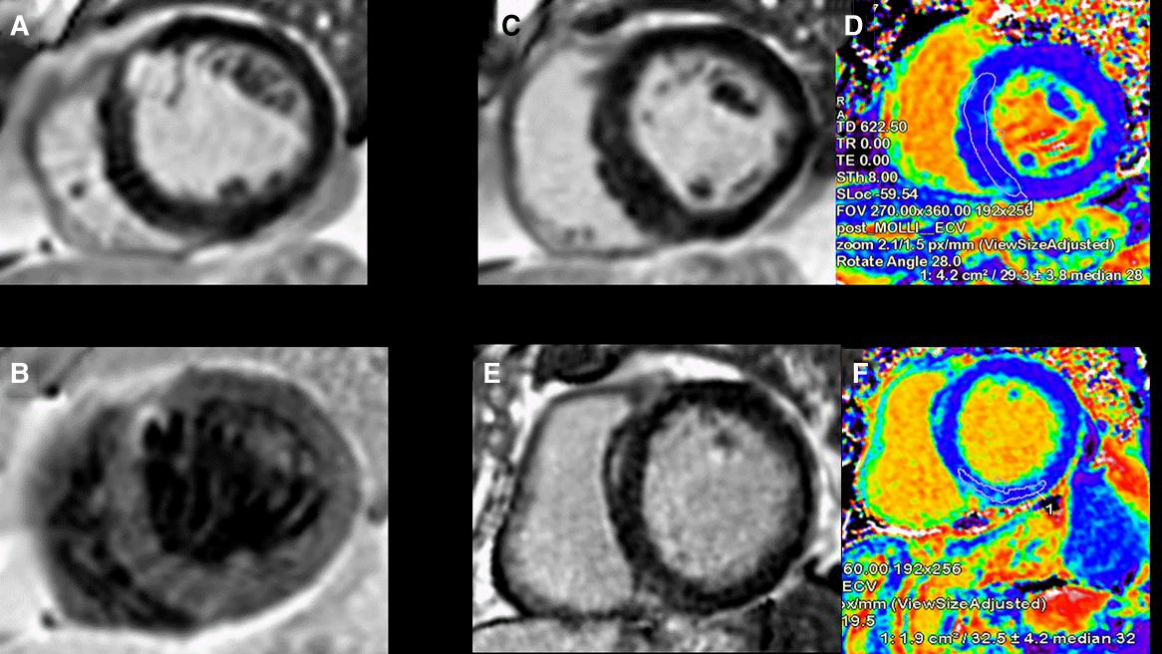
Patterns of myocardial fibrosis seen by magnetic resonance imaging in patients with chronic aortic regurgitation. (*A*) and (*B*) Discrete subendocardial infarction at mid anteroseptum; (*C*) and (*D*) increased wall thickness, but normal left ventricular mass, and no replacement myocardial fibrosis. Extracellular volume fraction (28%) is upper normal; (*E*) and (*F*) increased wall thickness with non-ischaemic midwall fibrosis at the basal anteroseptum and elevated extracellular volume fraction (32%), supportive more advanced left ventricular remodelling.
